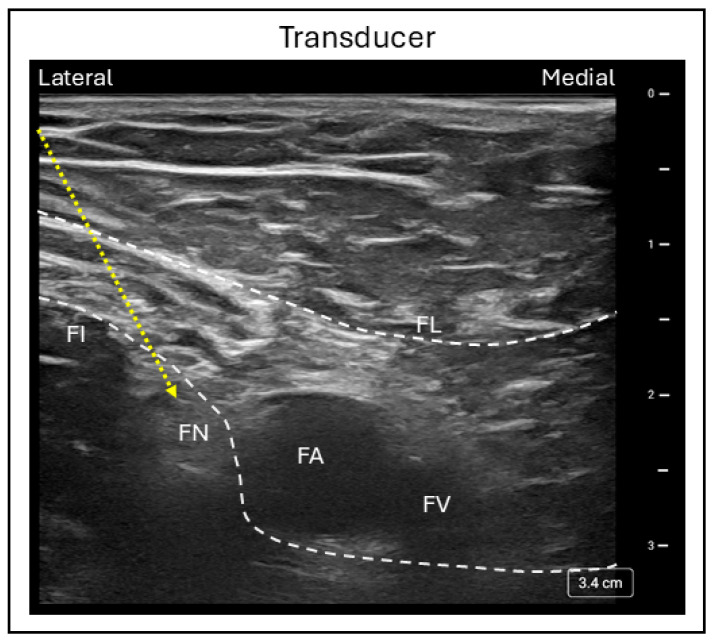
Ultrasound image demonstrating the in-plane femoral nerve block technique. FI = Fascia iliaca; FL = Fascia lata; FN = Femoral nerve; FA = Femoral artery; FV = Femoral vein; Dashed line = Needle trajectory.
